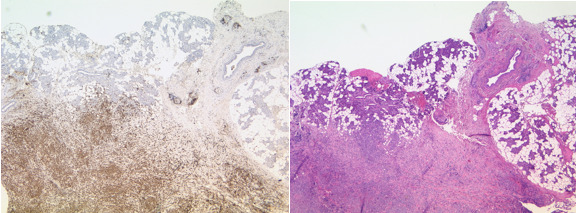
Intracranial expansion of the tumor due to perineural infiltration of the trigeminal nerve (4 × 1.8 cm) in a coronar T1‐MRI with contrast
